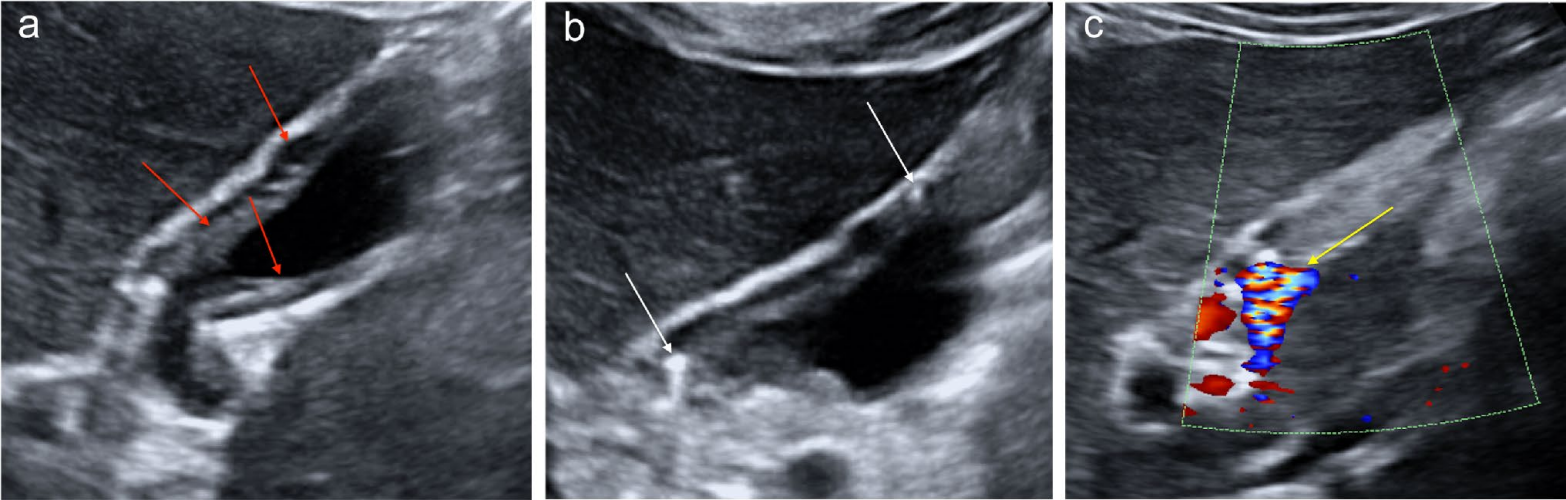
a The sonographic images of the diffuse adenomyomatosis type show thickening and irregularity with cholesterol crystals or calcifications along the whole gallbladder wall generating comet-tail reverberation artifacts (white arrows). b The sonographic images of the diffuse adenomyomatosis type show thickening and irregularity with cholesterol crystals or calcification along the whole gallbladder wall generating twinkling artifacts on the colour Doppler exam (yellow arrow). c The sonographic images of the diffuse adenomyomatosis type show thickening and irregularity (red arrows)
